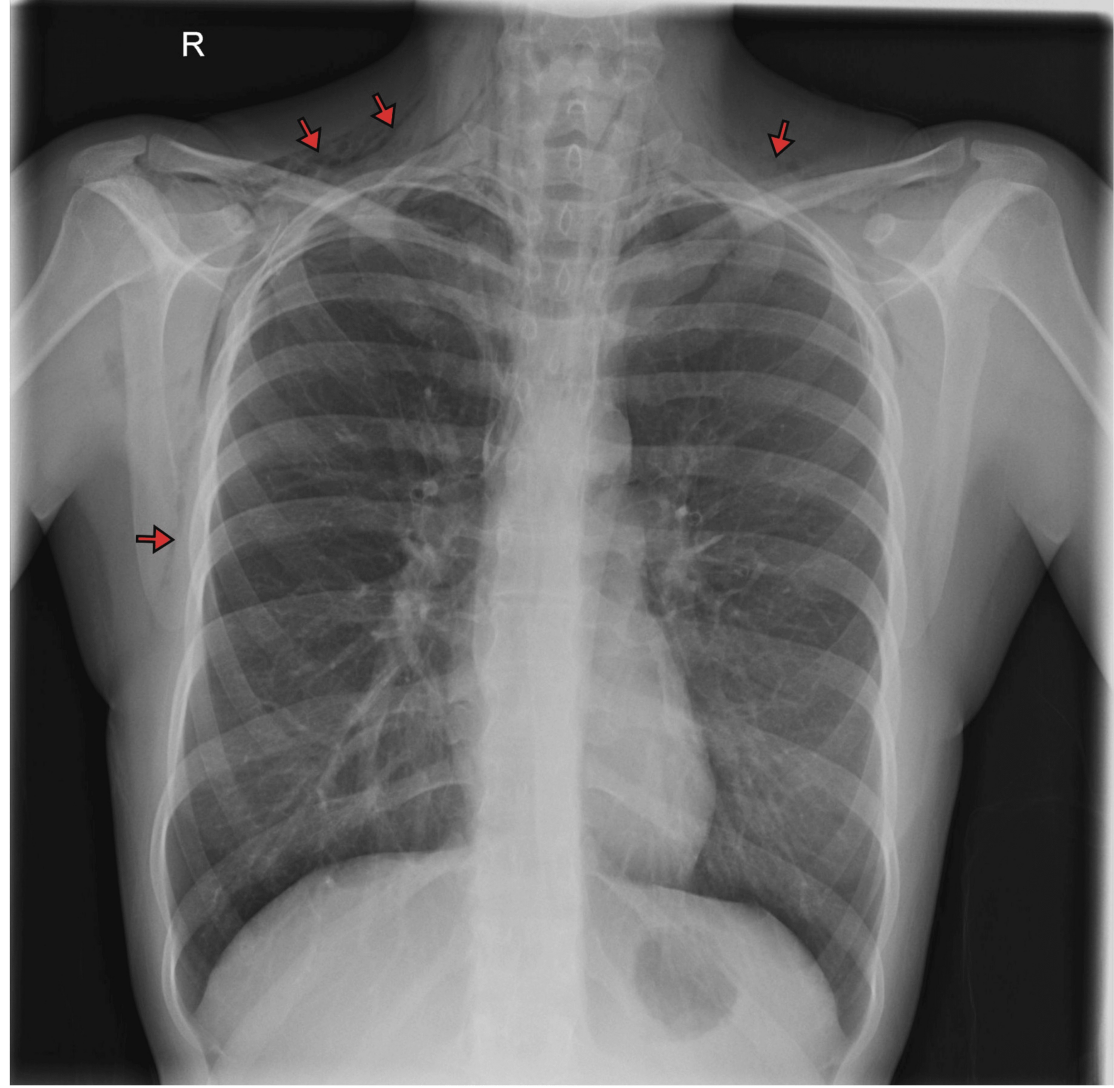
Chest radiograph showing subcutaneous emphysema This posteroanterior chest radiograph demonstrates extensive subcutaneous emphysema, visible as radiolucent streaks of gas (highlighted by red arrows) within the soft tissues of the supraclavicular region, lower neck, and axilla.
